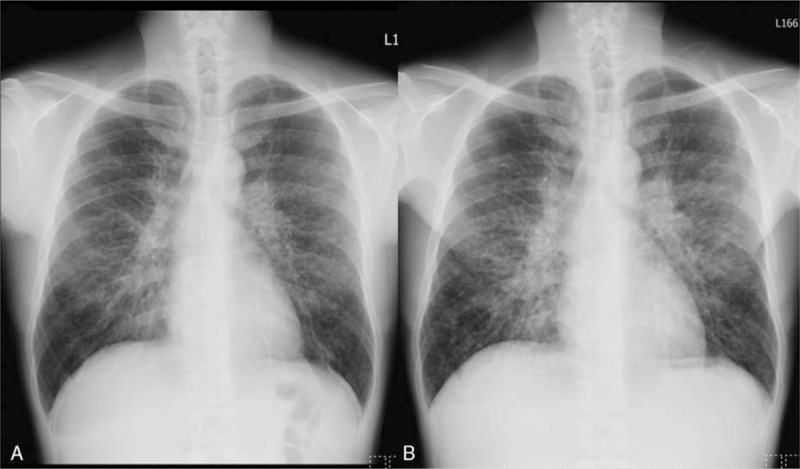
(A) Initial chest radiograph showing bilateral hilar enlargement and diffuse reticular-nodular pattern with central predominance. (B) Chest radiograph 4 years later showing progression of bilateral diffuse reticulo-nodular pattern.
